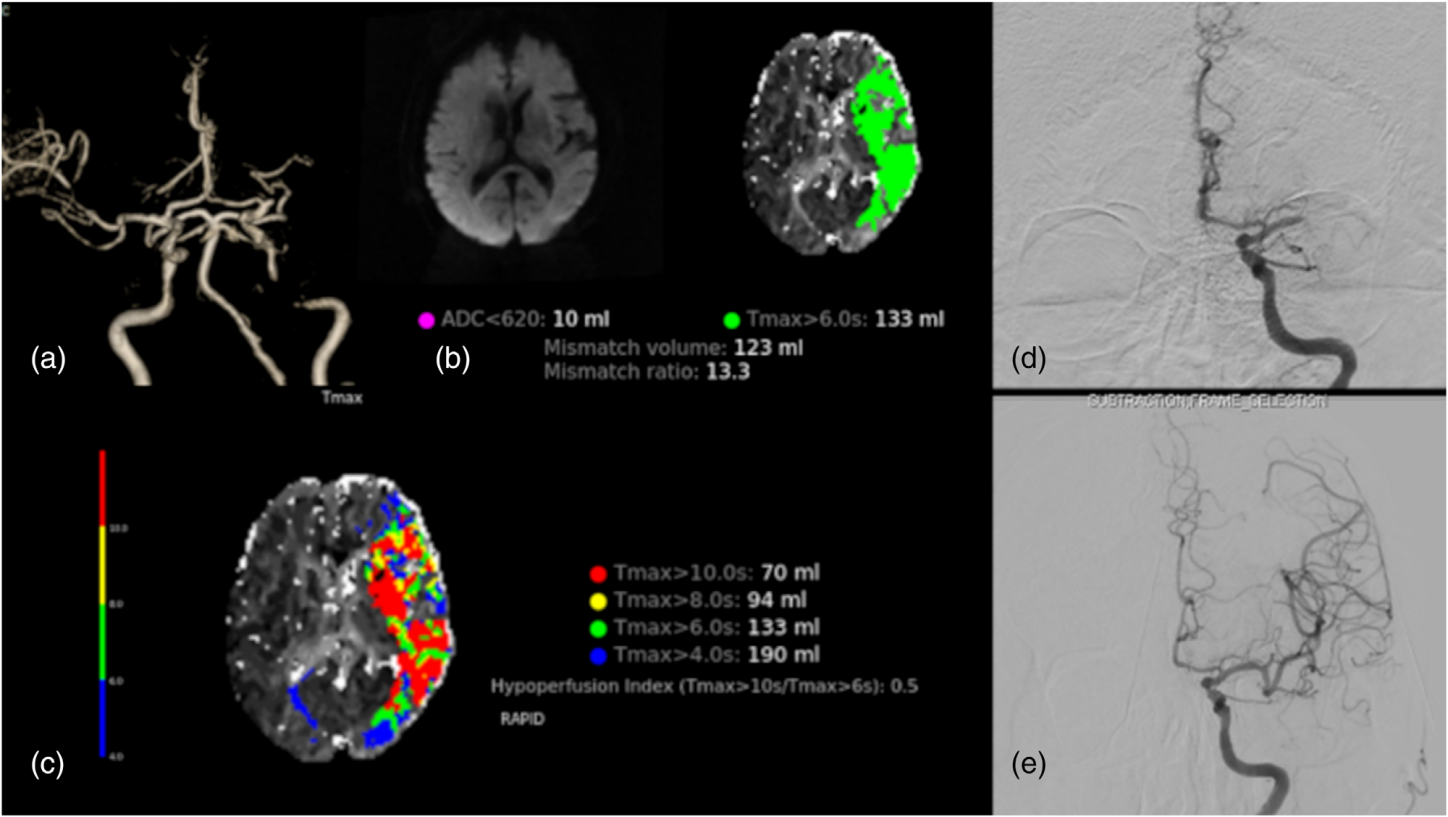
(A) MR angiography. (B) DWI‐PWI map. (C) *T*max map. (D) Angiography before endovascular treatment. (E) Angiography after endovascular treatment.
